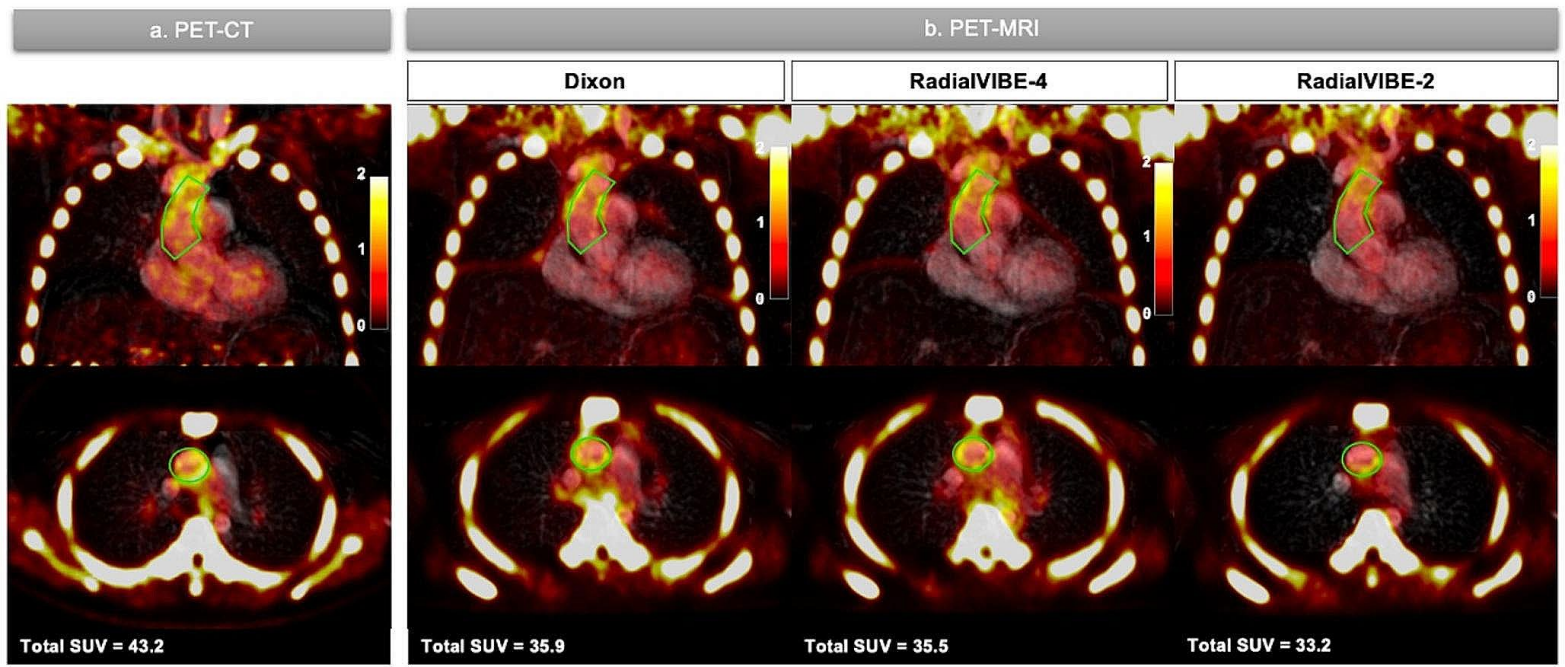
Representative coronal and axial images of ascending aortic sodium [^18^F]fluoride quantification in positron emission tomography (PET) images of a patient with bicuspid aortic valve. (a) PET-computed tomography (CT) co-registered with magnetic resonance angiography (MRA). (b) PET-magnetic resonance imaging (MRI) combined with MRA in each attenuation correction method (Dixon, RadialVIBE-4 and RadialVIBE-2). Green line represents region of interest around the ascending aorta. Colour scale on the right of images represents standardised uptake values. Total SUV = total standardised uptake value
